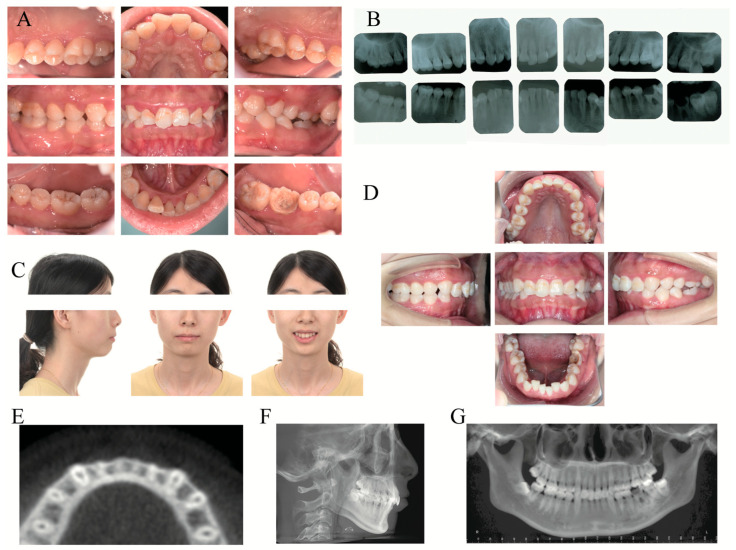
Clinical photographs and radiographs before periodontal treatment and orthodontic treatment. (A) Intraoral photographs before periodontal treatment. (B) Full-mouth periapical films. (C) Extraoral photographs before orthodontic treatment. (D) Intraoral photographs before orthodontic treatment. (E) Horizontal plane in cone-beam computed tomography (CBCT) at the level of 8 mm apical to CEJ of #21. (F) Lateral cephalogram. (G) Panoramic radiograph.
